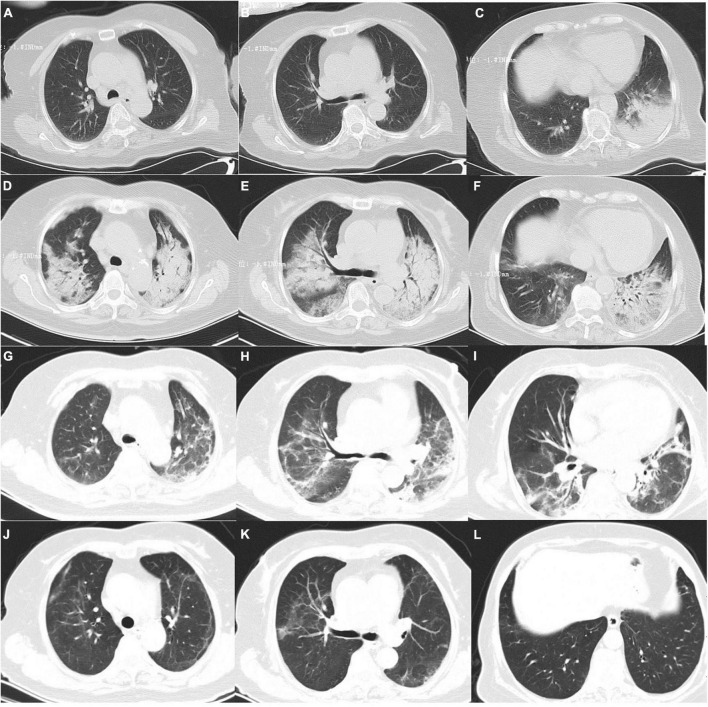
Patient’s chest CT scans: panels (A–C) show CT scans at the time of the patient’s first day of illness (November 7) in the local hospital. Panel (D–F) show CT scans on the 10th day of illness (November 17) in the local hospital. Panel (G–I) show CT scans on the 10th day after admission (November 27). Panel (J–L) show CT scans on the 26th day after admission (December 12).
